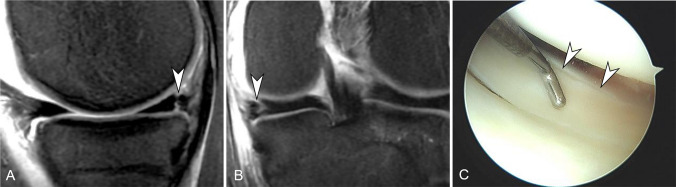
Longitudinal-vertical tear in a 15-year-old boy with ACL tear. Sagittal T2-weighted fat-suppressed MRI (A), coronal proton-density-weighted fat-suppressed MRI (B), and arthroscopic view (C) show complete longitudinal-vertical tear (arrowheads) of the posterior horn of the medial meniscus
